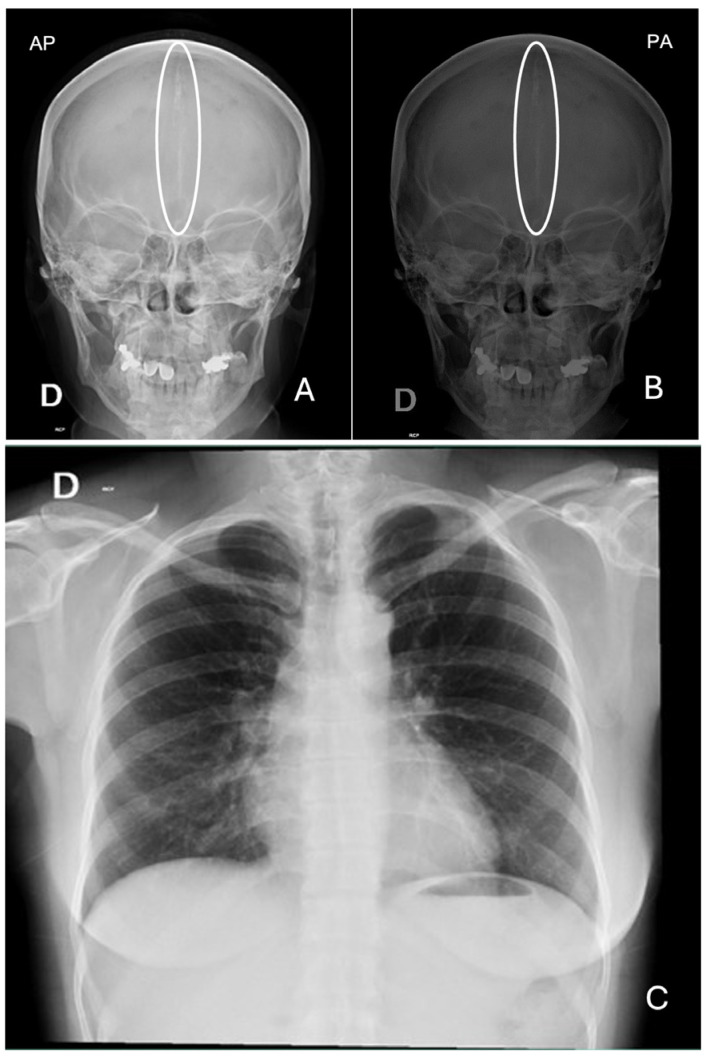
(A) Anteroposterior and (B) posteroanterior projection of skull X-ray showing a radiopaque area in the midline corresponding to falx cerebri calcification. (C) Chest X-ray, in which no alterations were identified.
